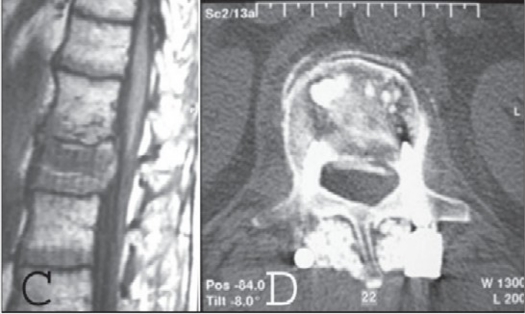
T1WI of preoperative MRI of same patient shows spinal canal narrowing at the level L_2_ (c) and postoperative CT scan (d) of the same patient at the level L_2_ showing calcibon on the anterior half of the vertebral body
